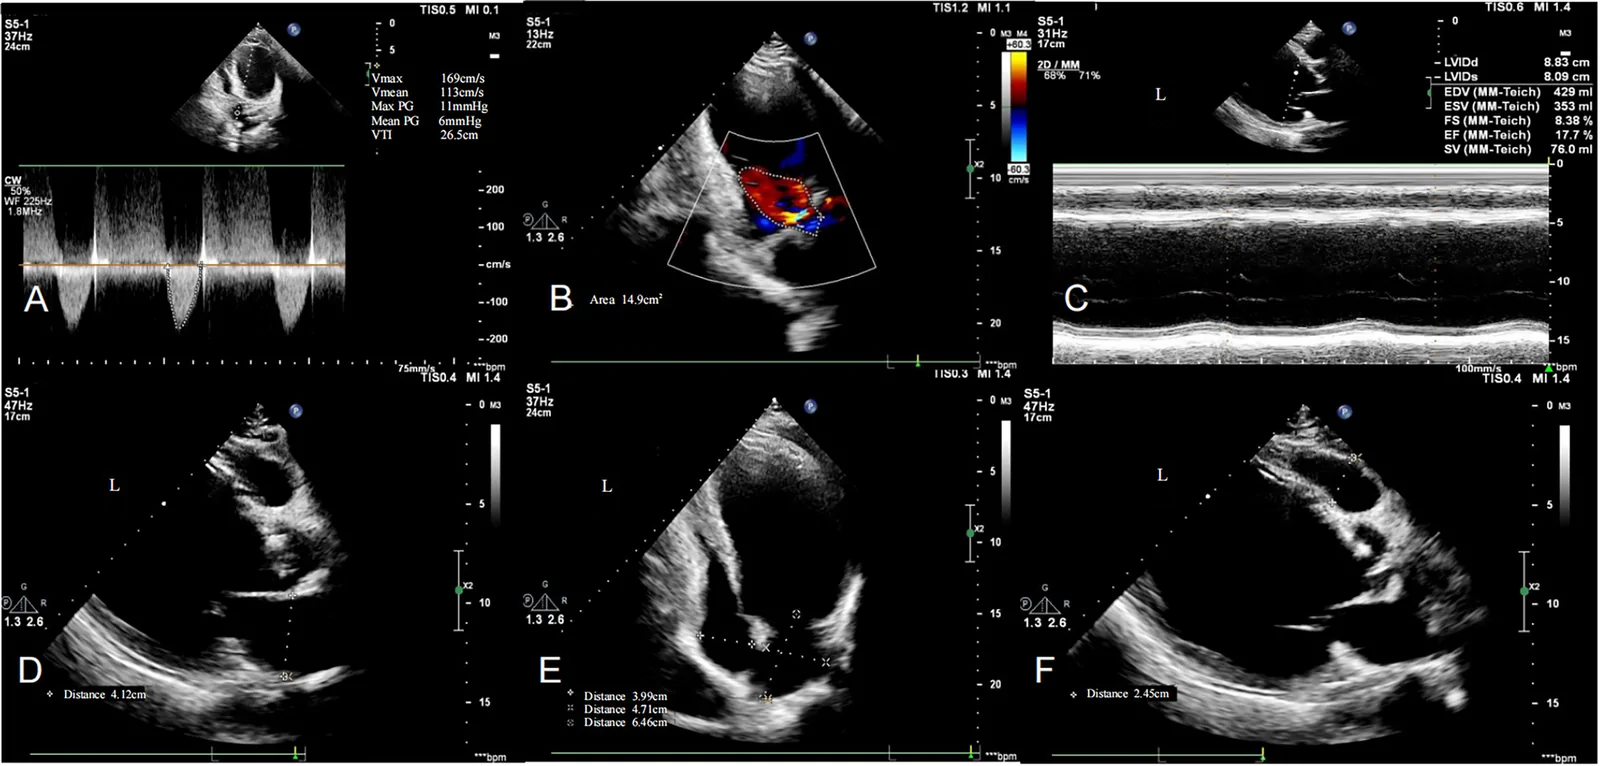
Preoperative echocardiography. (A) Aortic valve velocity 169 cm/s, peak pressure gradient 11 mmHg, mean pressure gradient 6 mmHg, velocity-time integral 26.5 cm; (B) Aortic regurgitation area 14.9 cm^2^; (C) Left ventricular end-diastolic diameter 88.3 mm, left ventricular end-systolic diameter 80.9 mm, ejection fraction 17.7%; (D) Left atrial anteroposterior diameter 41.2 mm; (E) Left atrial transverse diameter 47.1 mm, left atrial longitudinal diameter 64.6 mm, right atrial transverse diameter 39.9 mm; (F) Right ventricular anteroposterior diameter 24.5 mm.
